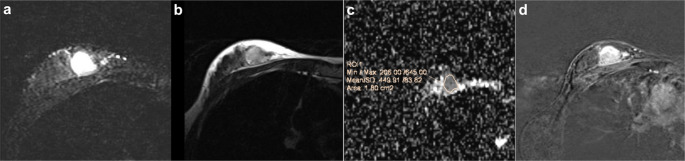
T-cell lymphoblastic leukemia in a 20-year-old woman. (a) Fat-suppressed and (b) non–fat-suppressed T2-weighted images demonstrate an oval-shaped T2-hyperintense mass with circumscribed margins in the right breast. (c) The apparent diffusion coefficient (ADC) map demonstrates marked diffusion restriction with very low ADC values, reflecting high tumor cellularity. (d) Axial postcontrast subtraction T1-weighted image reveals heterogeneous enhancement pattern
